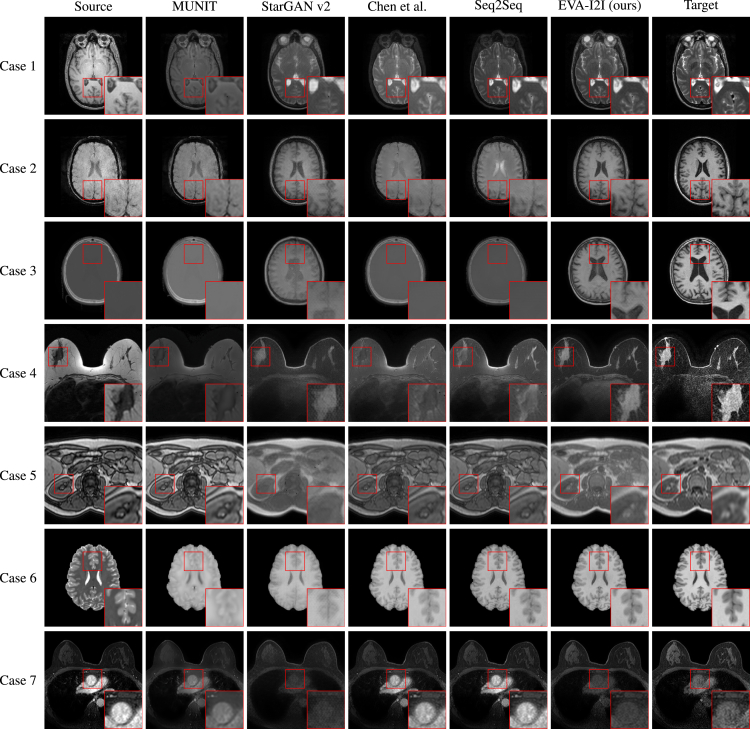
Axial visualization of translated images generated by comparison methods Cases 1–5 from the in-domain datasets show the transformation from T1 to T2, susceptibility weighted imaging (SWI) to T1, CT to T1, non-fat saturated to fat saturated, and out of phase to in phase. Case 6 and case 7 from the out-domain datasets show the transformation from T2 to T1 and post-contrast dynamic contrast-enhanced (DCE) to pre-contrast DCE. Our EVA-I2I attains better quality and a style that resembles the target image.
